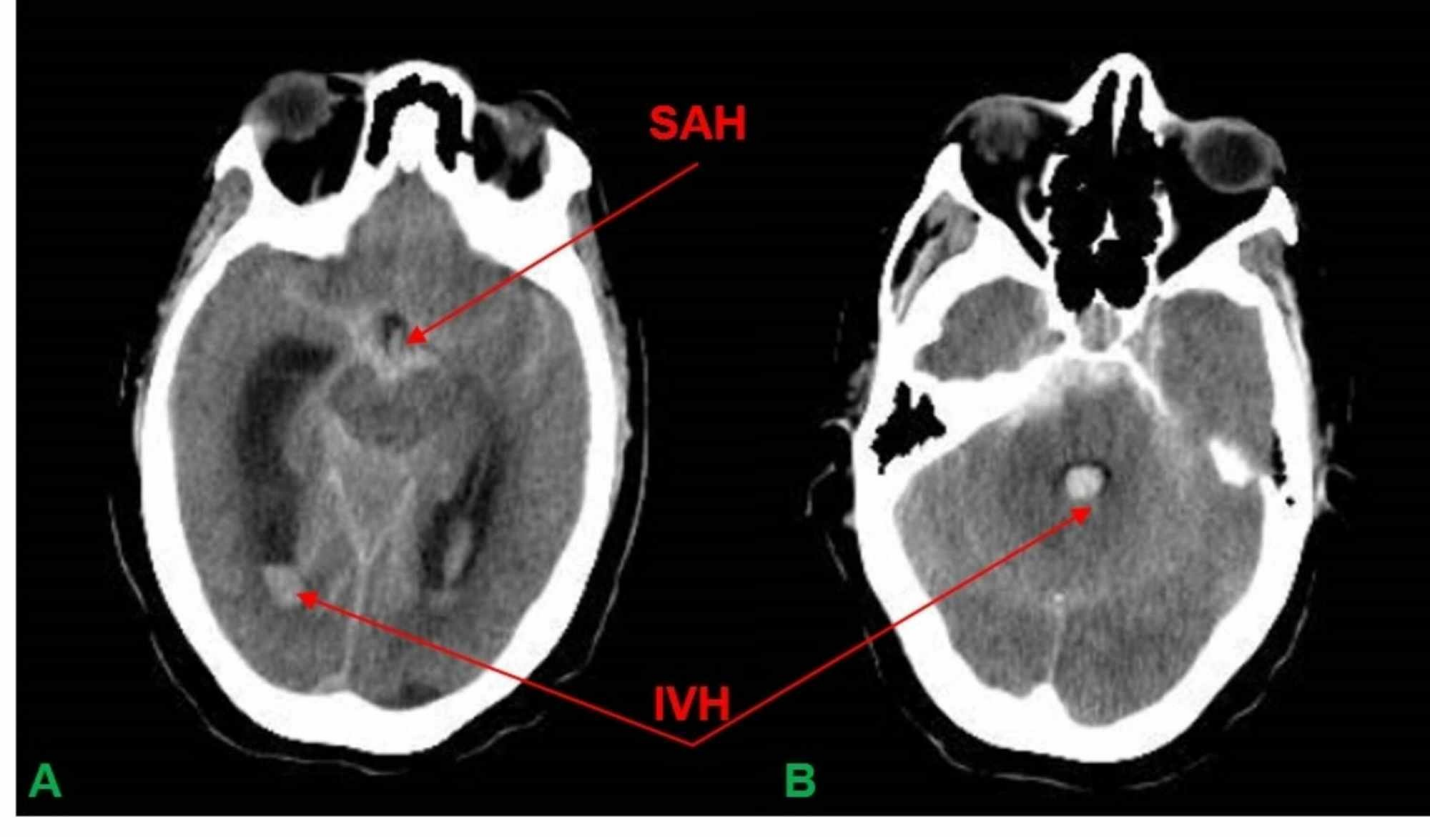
A. CTH revealing diffuse SAH with IVH. B. CTH revealing IVH CTH = CT Scan of Head; SAH = Subarachnoid Hemorrhage; IVH = Intraventricular Hemorrhage
